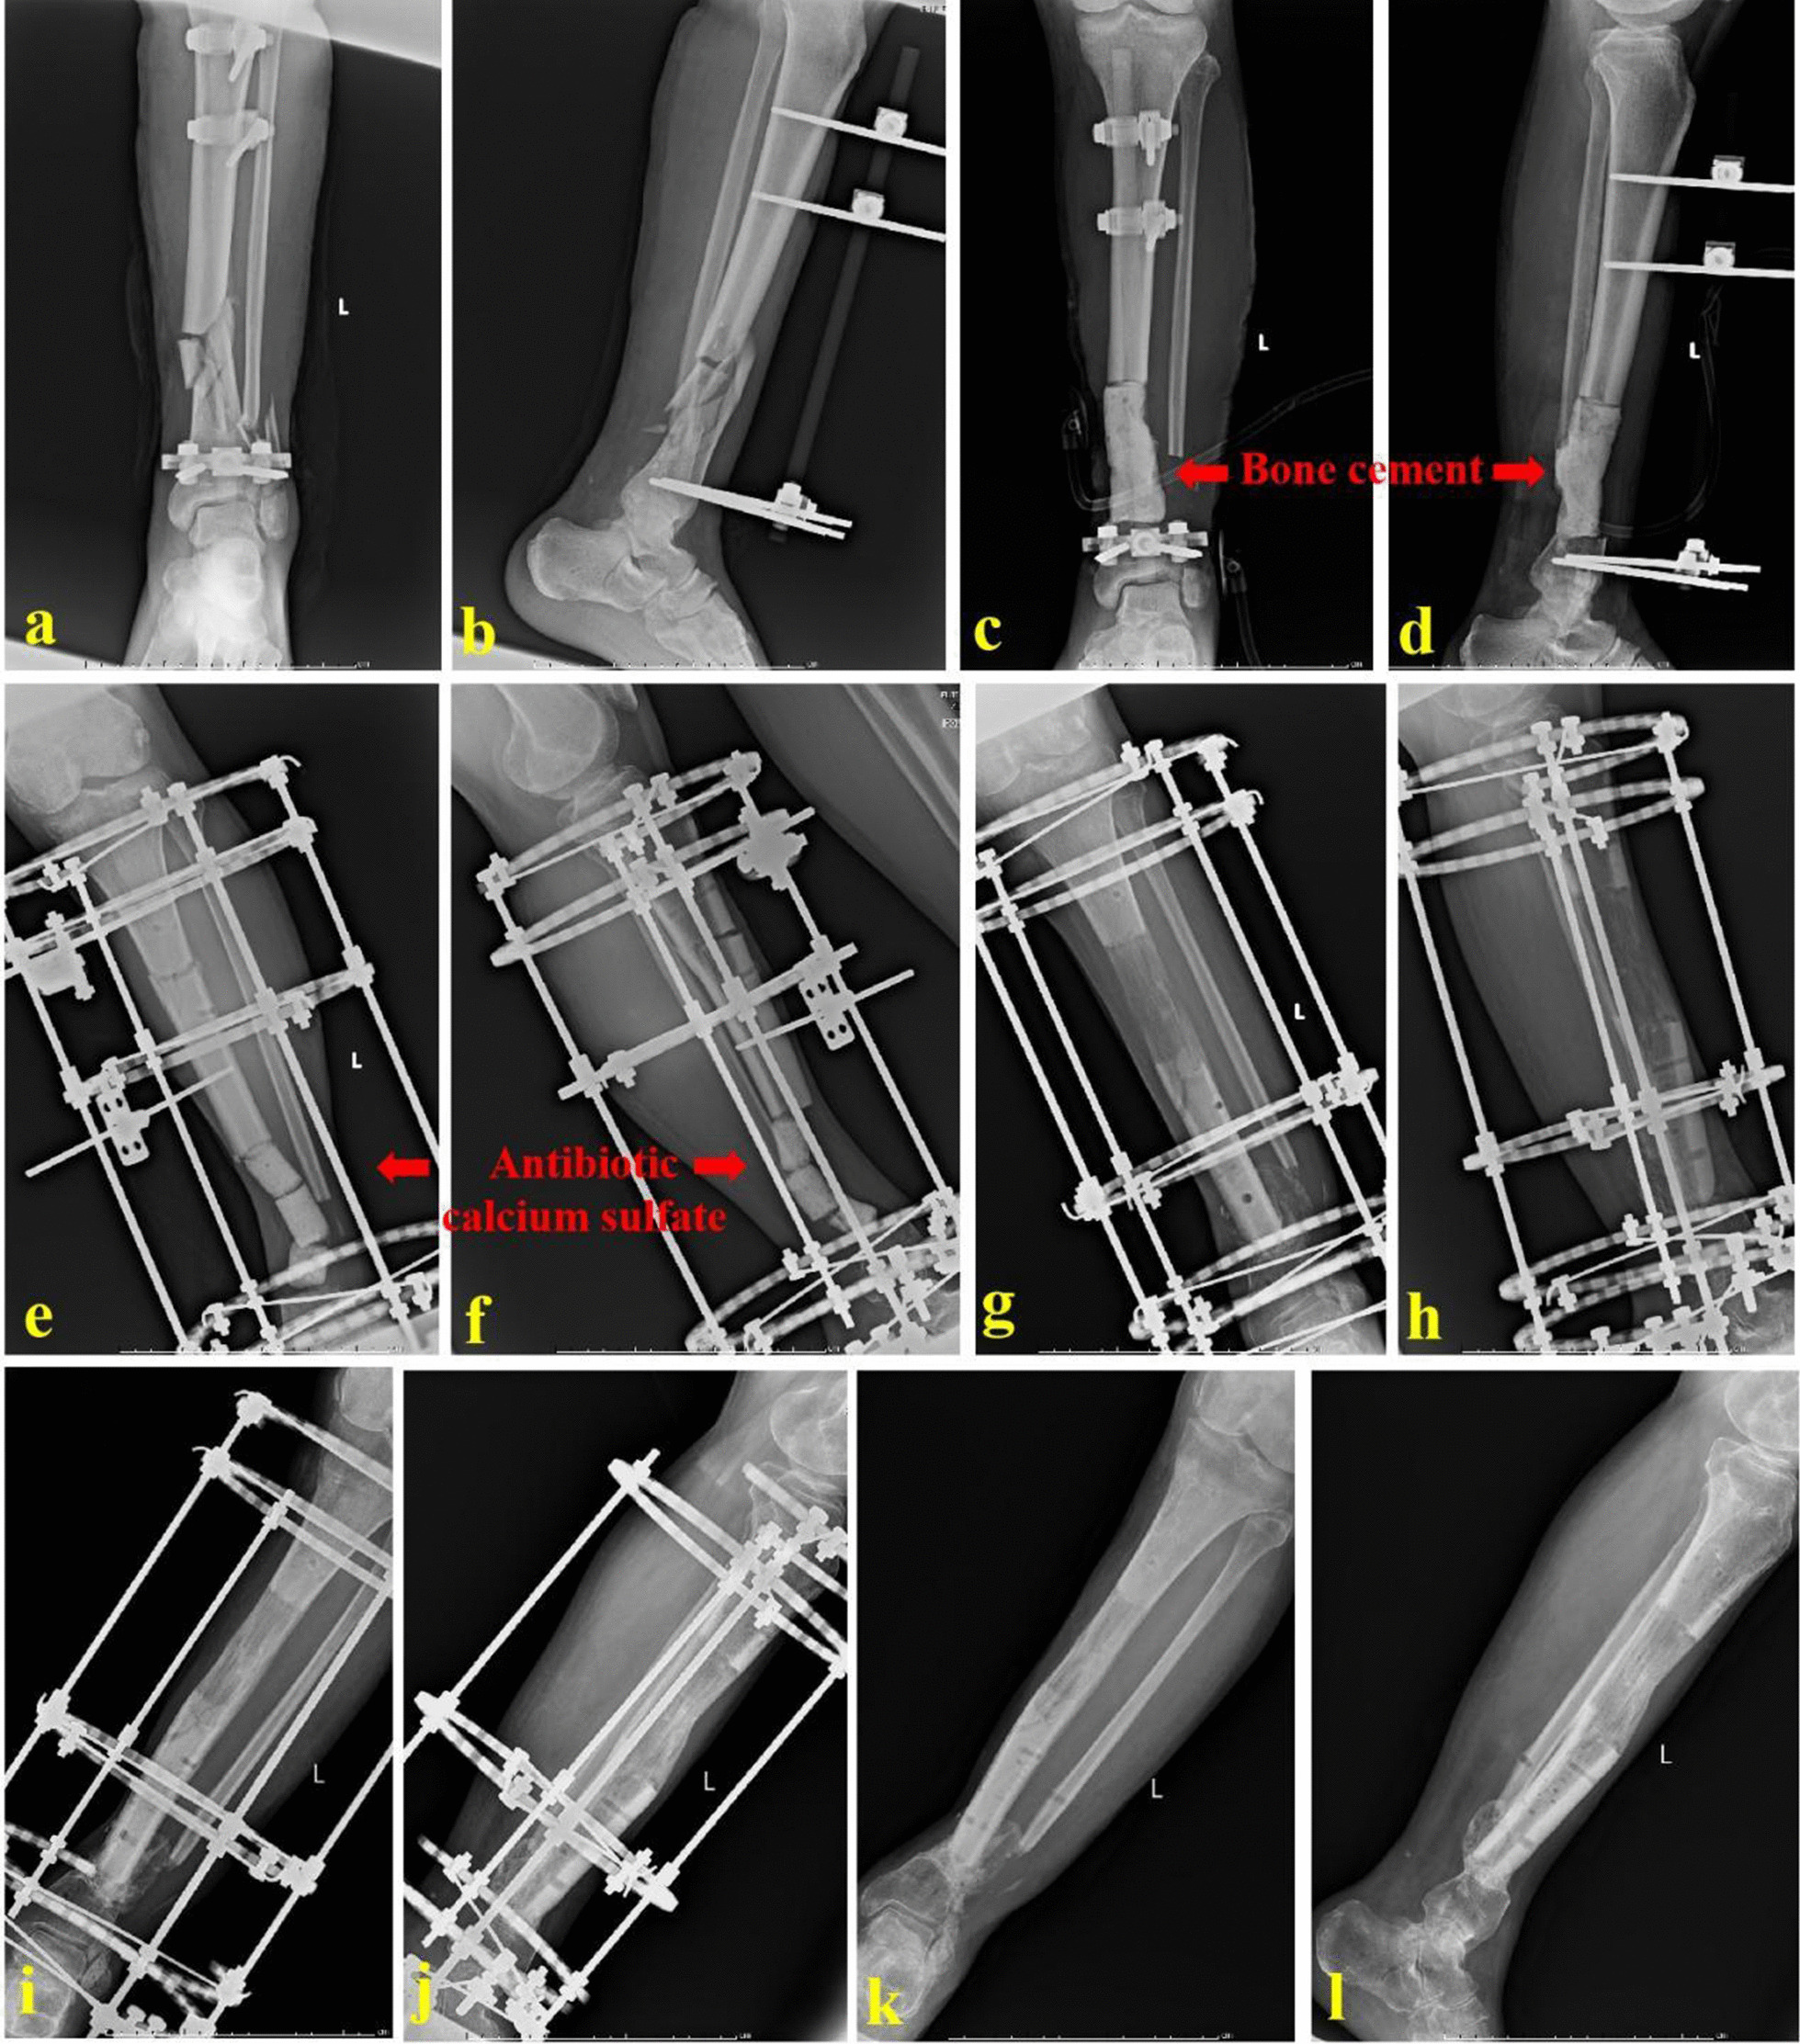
A 52-year-old male was successfully treated by ACSBT technique. a, b The patient suffered from a severe open fracture and infection of the tibia; c, d after thorough debridement, bone cement was filled into the bone defect site. The tibial defects were 9.0 cm; e, f the Ilizarov annular transport frame was installed, and antibiotic-loaded calcium sulfate was inserted; g–j ACSBT technique was successfully applied to repair the tibial defects; and k, l X-ray images at one year after removing the annular frame. ACSBT stands for antibiotic calcium sulfate-loaded bone transport
